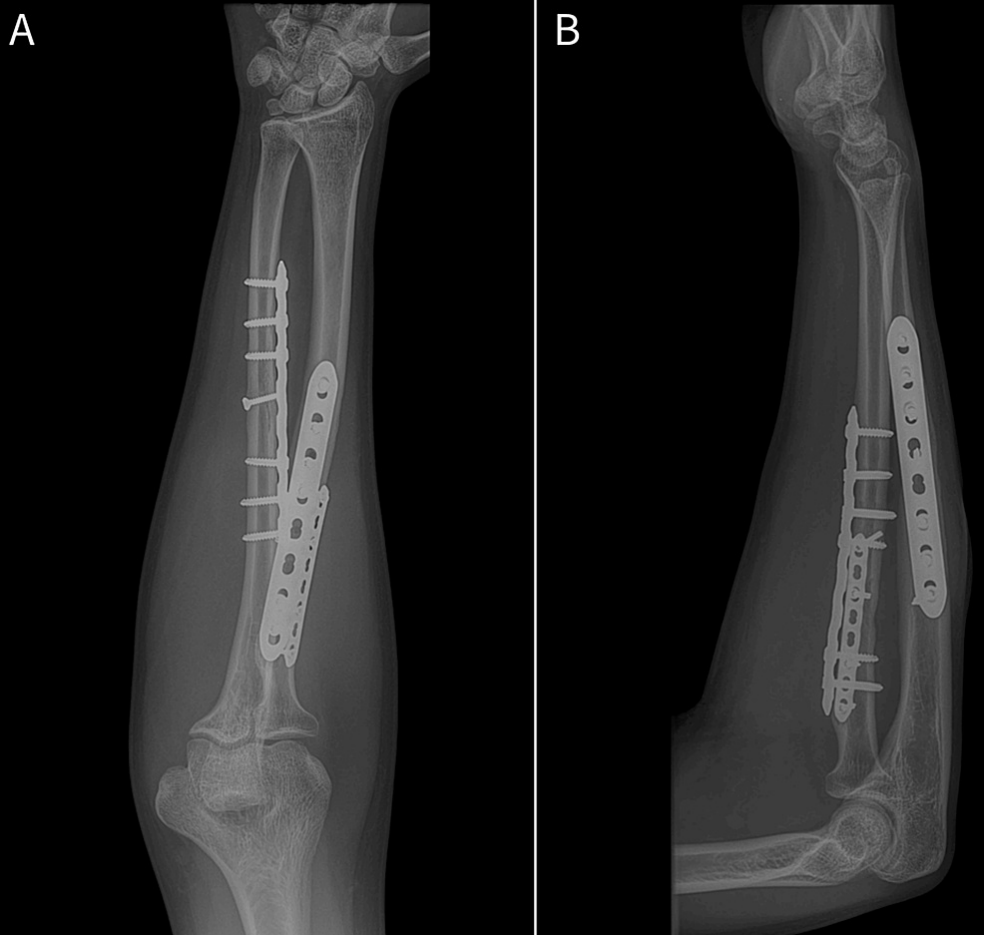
Radiographic union 12 weeks postoperatively A) Anteroposterior forearm radiograph; B) lateral forearm radiograph
